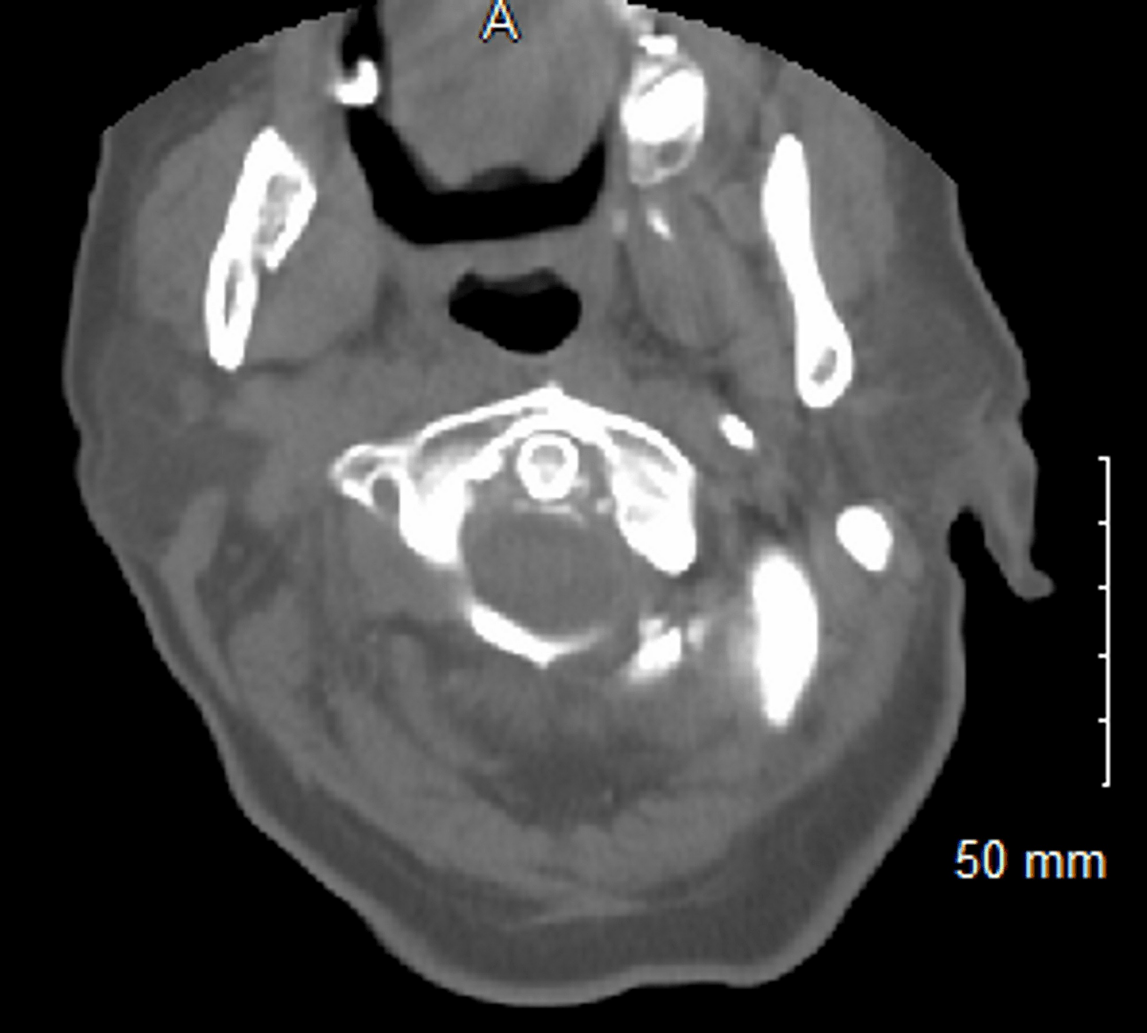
Cervical spine CT scan showing calcifications of the transverse and alar ligament (crowned dens syndrome)
